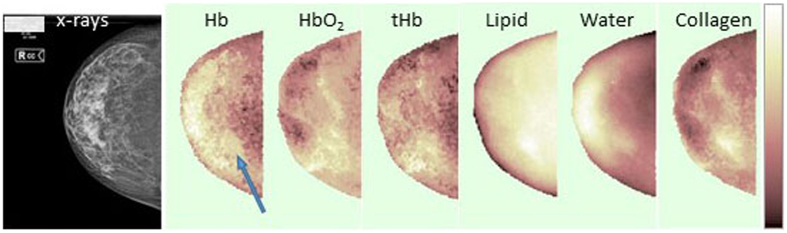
Example of tissue composition maps. Right cranio-caudal view of the patient #117 with a fibroadenoma (10 mm size) in the lower-outer quadrant. From left to right, x-ray image and Δ*C*_*i*_ maps of the main breast constituents (*Hb, HbO*_*2*_, *tHb*, lipid, water and collagen). A blue arrow points to the lesion location. The color-bar range for Δ*C*_*i*_ maps is: −15 to 30 (Δ*Hb* (μM)), −15 to 40 (Δ*HbO*_*2*_ (μM)), −10 to 65 (Δ*tHb* (μM)), −99.5 to 31.5 (ΔLipid (mg/cm^3^)), −200.0 to 325 (ΔWater (mg/cm^3^)), −70 to 140 (ΔCollagen (mg/cm^3^)).
